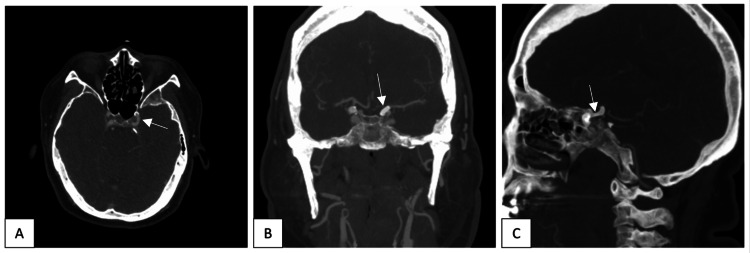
Axial (A), coronal (B), and sagittal (C) CT angiograms post-flow diverters insertion with no evidence of subdural hemorrhage
